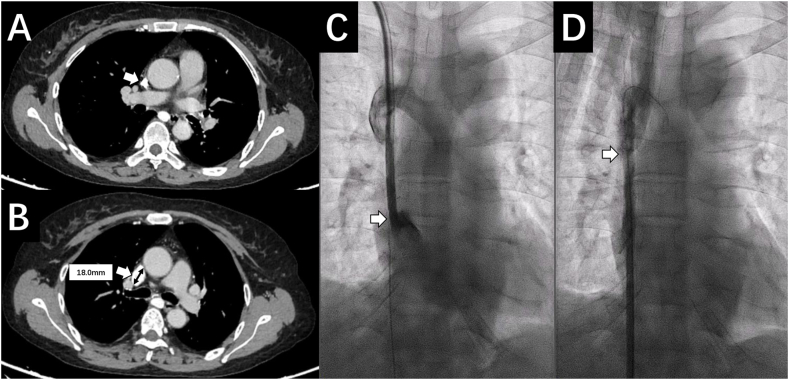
A: Superior vena cava calcification and stenosis by CT venography, white arrow: superior vena cava calcification and stenosis. B: Measurement of superior vena cava diameter, white arrow: superior vena cava diameter was estimated to be about 18.0 mm. C: Superior vena cava stenosis by DSA, white arrow: proximal remnant of superior vena cava. D: Superior vena cava stenosis by DSA, white arrow: distal remnant of superior vena cava.
